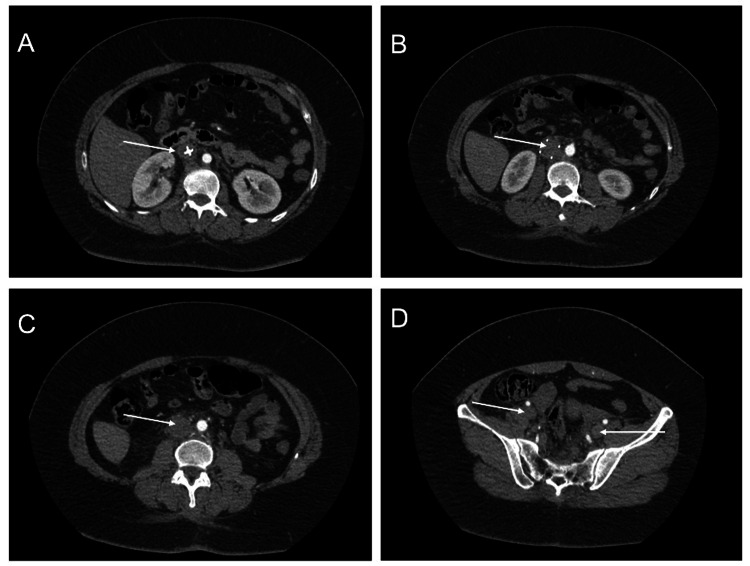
CT angiography demonstrating IVCF thrombosis extending down to the bilateral iliac veins. A) IVCF head. B) Thrombosed IVCF. C) Thrombosis of IVC. D) Thrombosis of bilateral iliac veins. CT: computed tomography; IVCF: inferior vena cava filter
